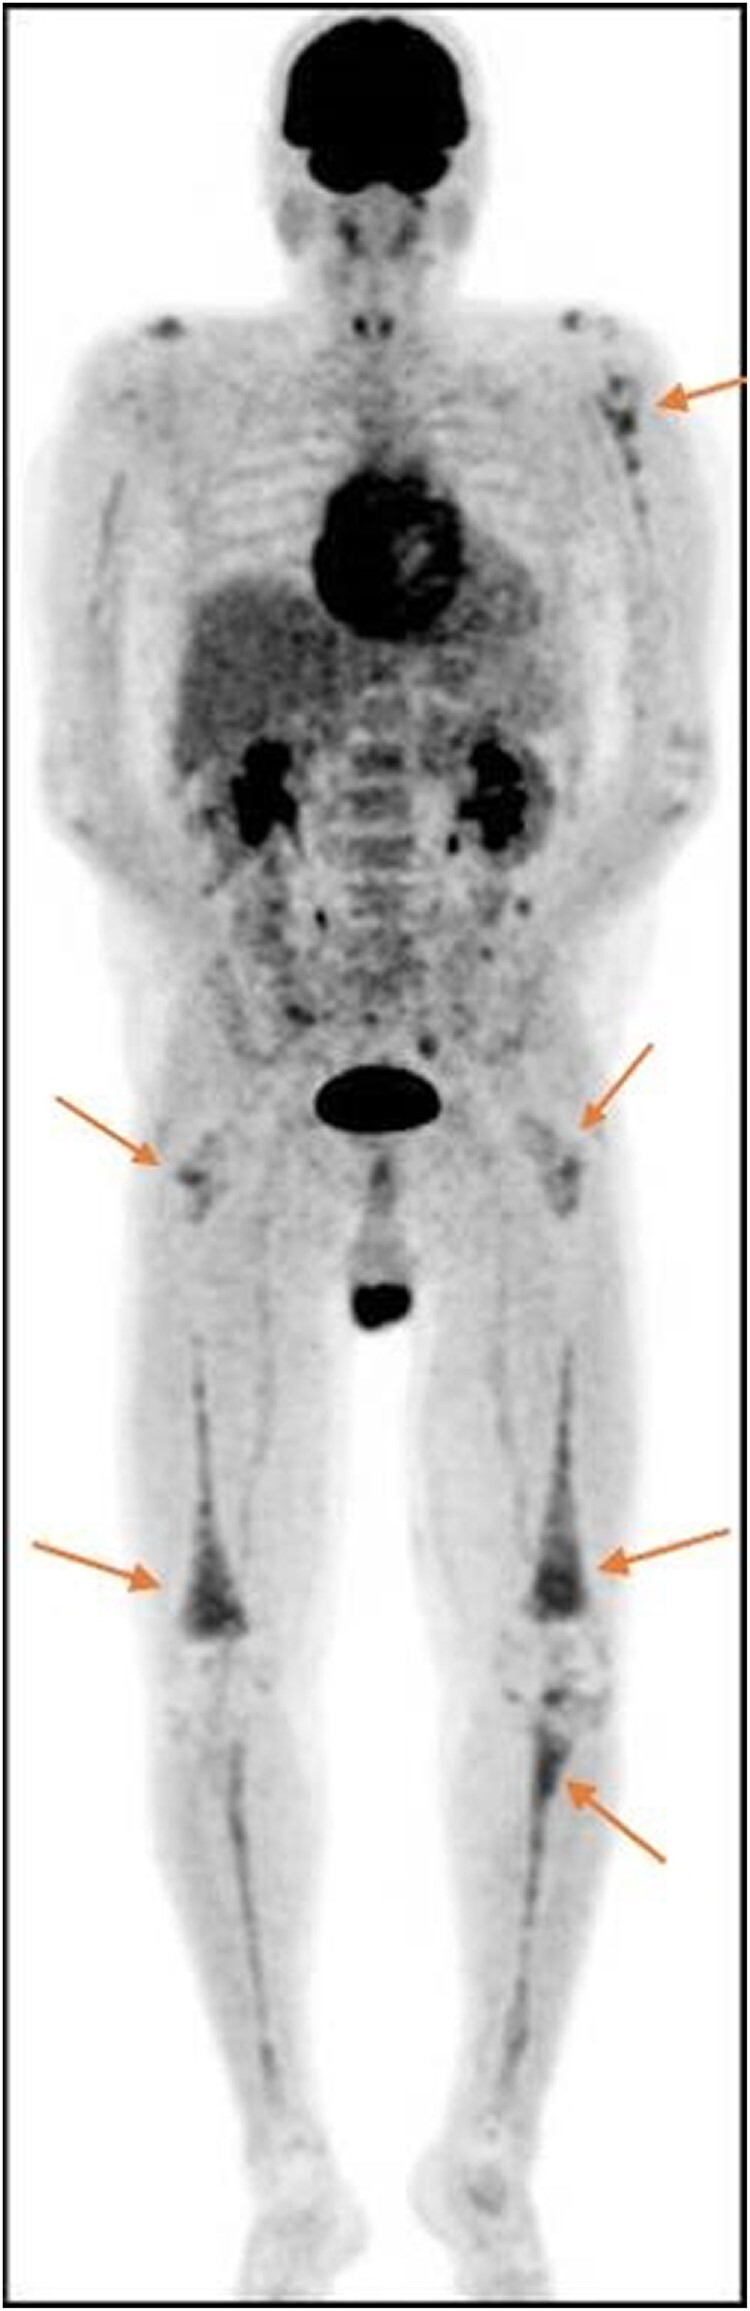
18F-FDG PET/CT demonstrates predominantly epiphyseal 18F-FDG–avid disease.
